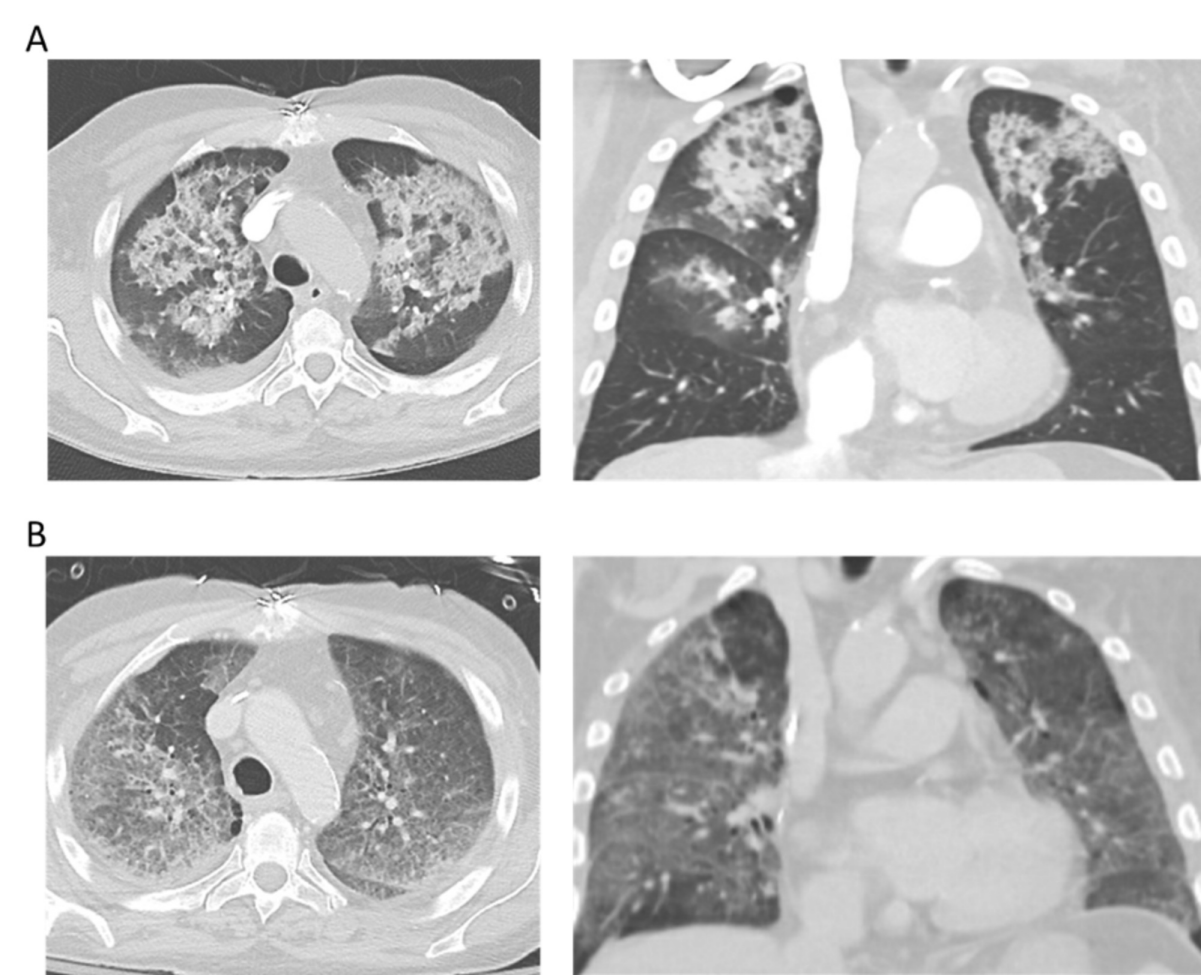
CT Angiogram of the Chest (A) Representative transverse (left) and coronal (right) images of the CT angiogram performed on hospital day 4 showing apical-predominant fluid-filled alveoli. (B) Representative images of the CT chest (with contrast) performed on hospital day 12 showing persistent ground-glass opacification of the lungs.
